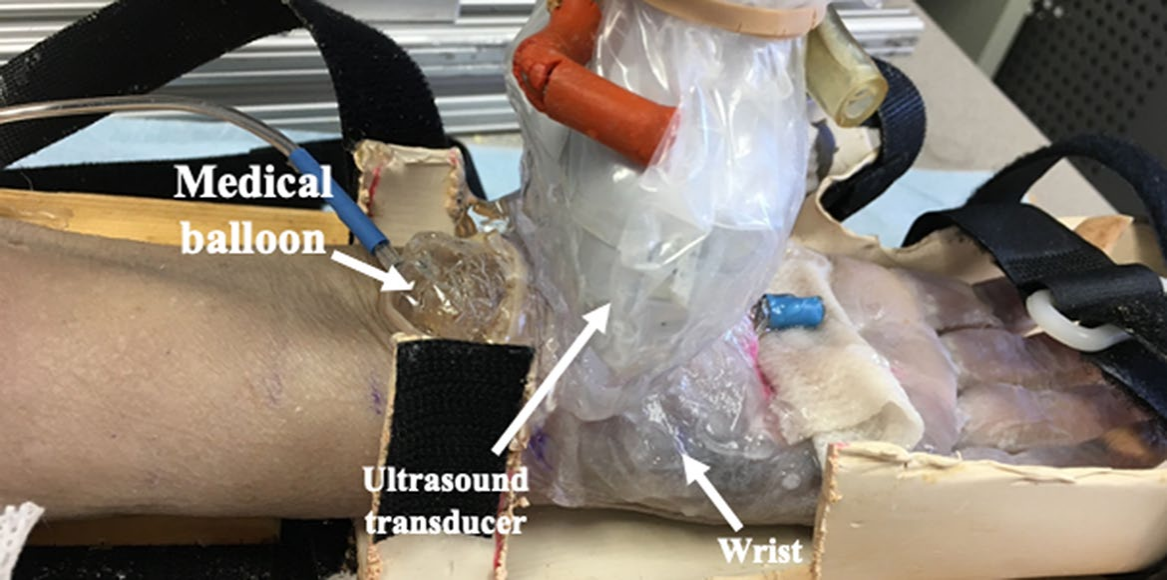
Experimental setup for carpal tunnel imaging. Note that the ultrasound transducer was encased in a specialized cover for hygiene consideration
